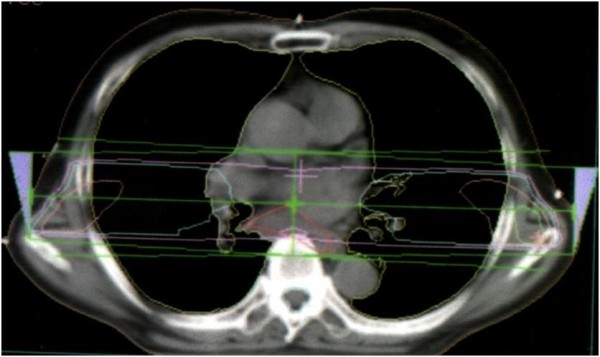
Chest CT image showing radiation dose distribution after 40 Gy.
